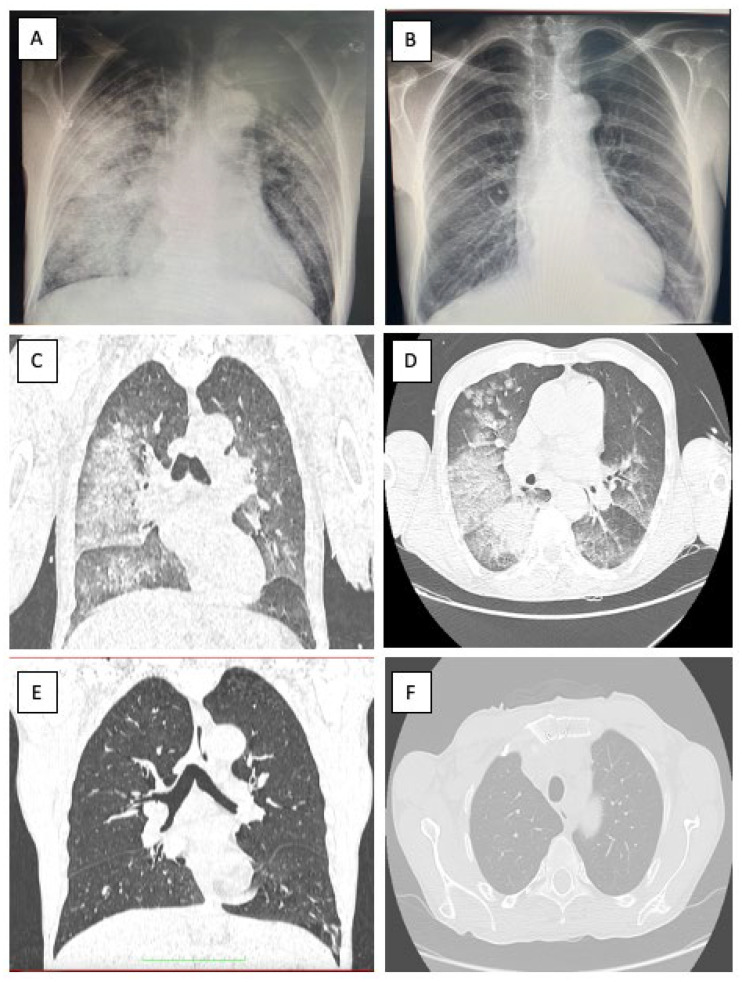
(A) Chest XR at presentation showing bilateral alveolar infiltrates, with both central and peripheric distribution in the inferior two-thirds of both lungs (more important on the right)—suggesting an infectious pulmonary disease; (B) chest XR at discharge showing resolution of pulmonary lesions; (C,D) computed tomography aspect of the lungs at presentation resembling multiple, bilateral areas of consolidation, with air bronchogram included and areas of ground-glass attenuation with a mainly central distribution, in the two-thirds of both pulmonary parenchyma (more significant in the right lung) suggesting an infectious pneumonia; (E,F) computed tomography aspect of the lungs at discharge with resolution of initial lesions.
